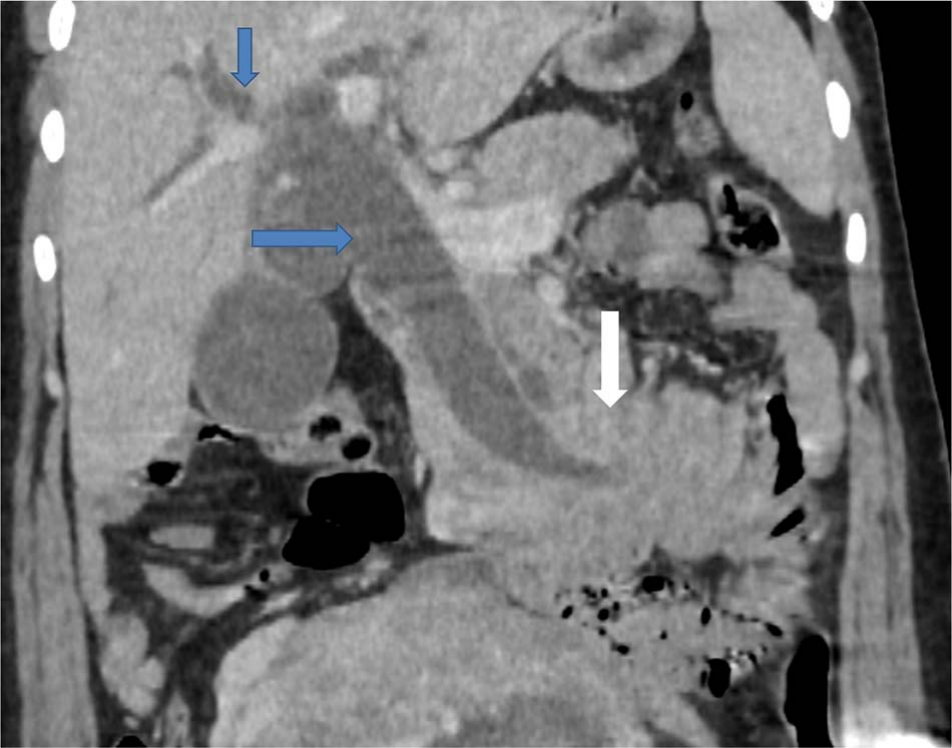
Coronal contrast-enhanced CT image showing the intussusception causing traction on the distal common bile duct (arrow at the bottom), resulting in significant upstream dilatation of the extrahepatic and intrahepatic bile ducts (two arrows at the top).
